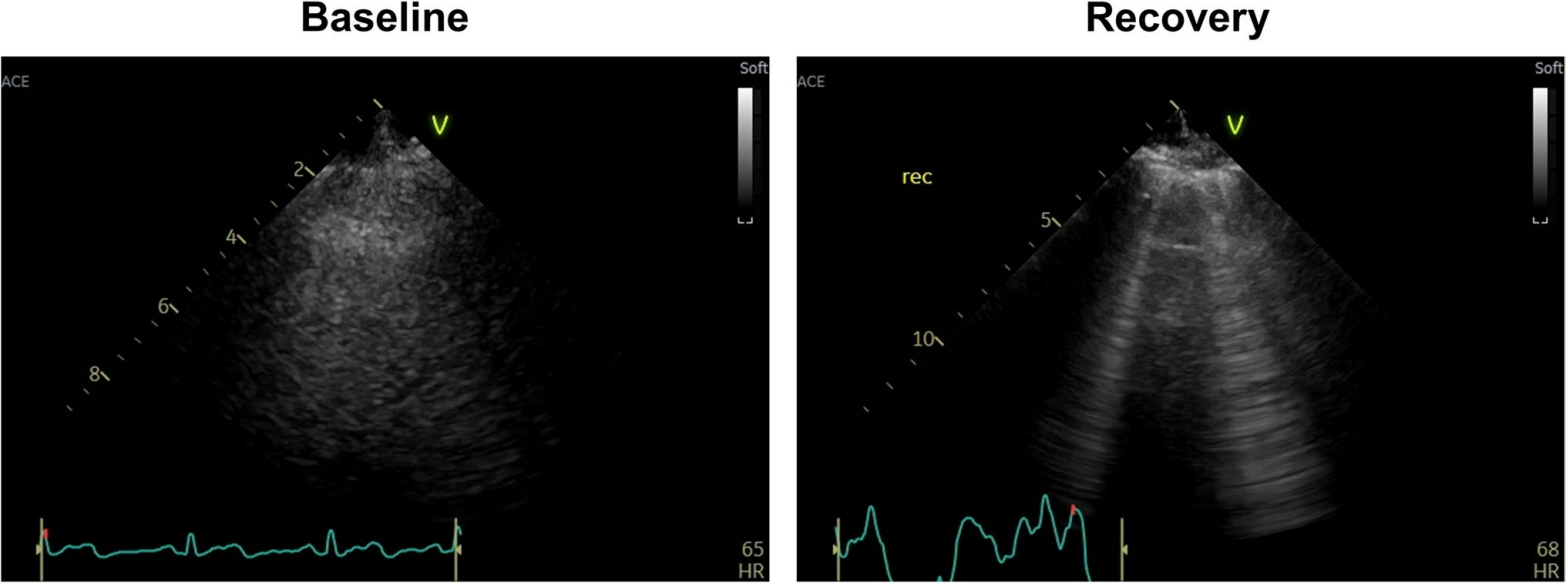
Lung ultrasound to identify ultrasound B-lines during exercise stress echocardiography. Ultrasound B-lines are absent at baseline but develop in the recovery phase in a patient with heart failure with preserved ejection fraction (HFpEF). Ultrasound B-lines are laser-like hyperechoic lines that originate from the pleural line and extend to the bottom of the ultrasound screen
